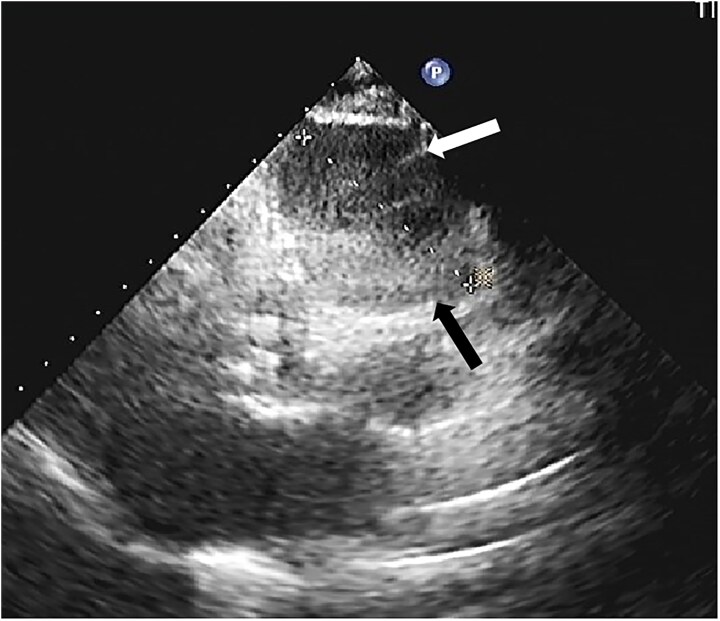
Transthoracic echocardiography revealed a tumour in the mediastinum (black arrow) with an anechoic area visible within the tumour (white arrow).
